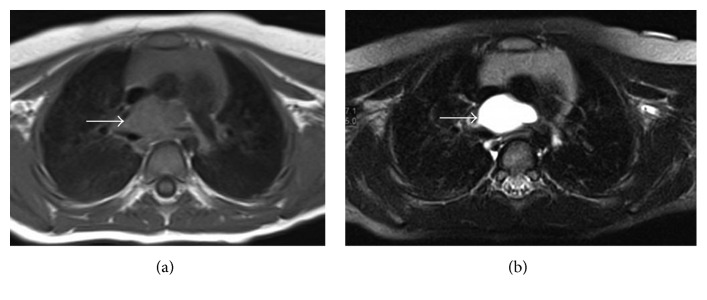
MRI of the chest showing lobulated mass lesion heterogeneously hypointense on T1-weighted images (arrow) (a), and hyperintense on T2-weighted images (arrow) (b). The mass is in the middle mediastinum, at the level of the carina and adjacent to the trachea extending to the subcarinal region, causing compression on the carina and both main bronchi, more pronounced on the left main bronchus.
